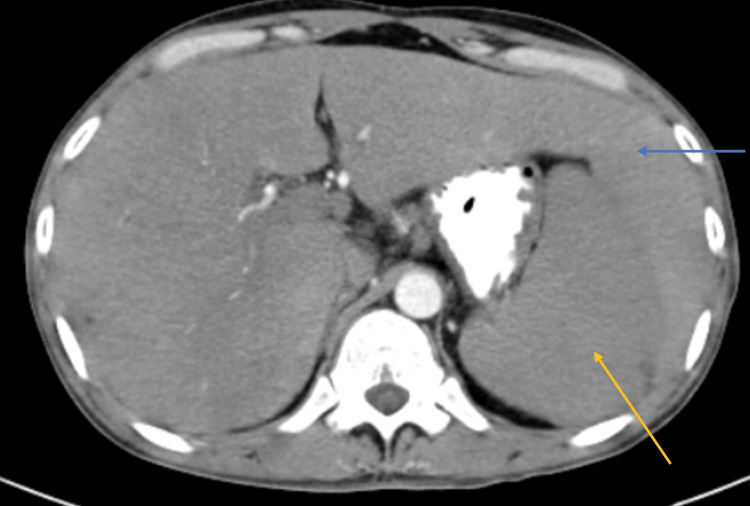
CT of the abdomen showing enlarged left lobe of the liver (blue arrow) enveloping the spleen (yellow arrow) CT: computed tomography
